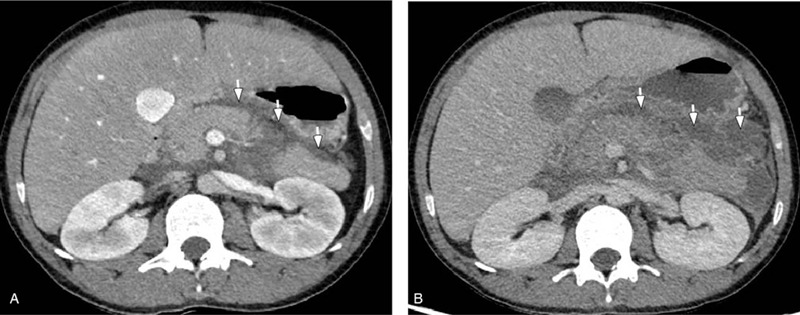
A: Abdominal contrast-enhanced CT showing edema and peripancreatic necrotic fluid collection (white arrows). B: Abdominal contrast-enhanced CT showing increased peripancreatic necrotic fluid collection (white arrows). CT = computed tomography.
